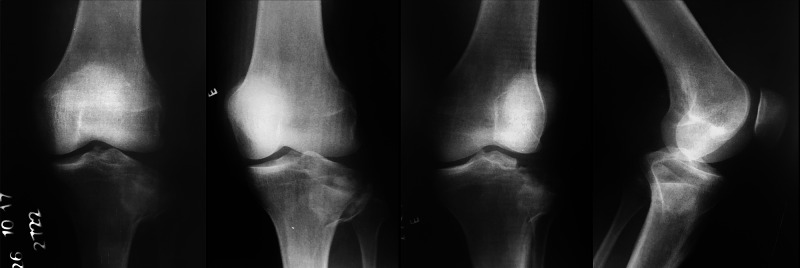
GM, 35-year-old, male patient, fall from a motorcycle Preoperative X-rays of the left knee showing a Kfuri-Schatzker type II A tibial plateau fracture
